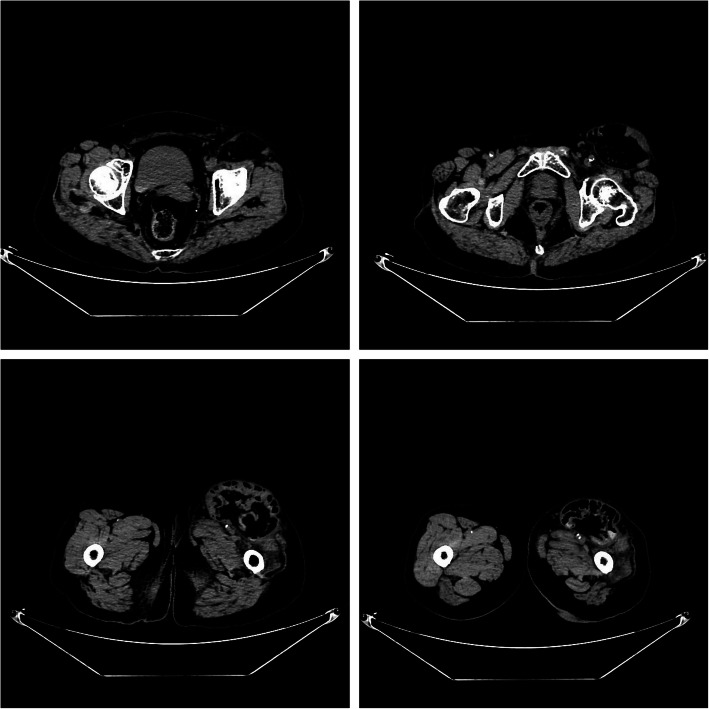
CT images of the lower abdomen and Thighs
